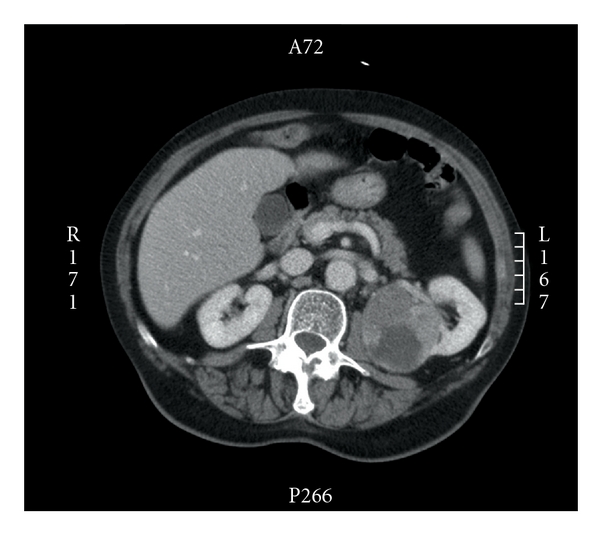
CT transversal image showing a 7 cm tumor in the upper pole of the left kidney with central necrosis.
